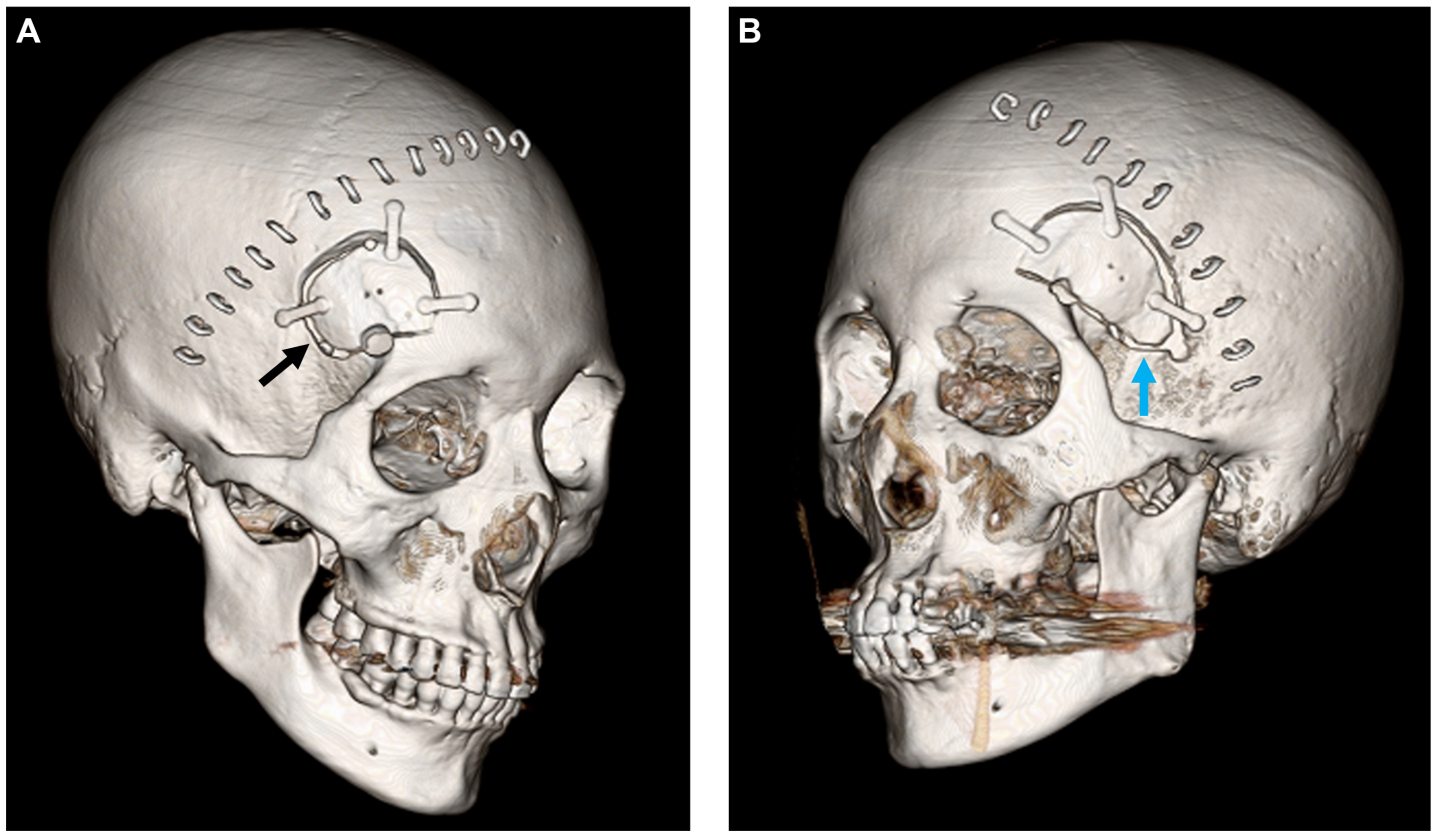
(A) Postoperative 3D CT image of lateral supraorbital craniotomy (black arrow). (B) Postoperative image of pterional craniotomy with sphenoid bone working (blue arrow).
